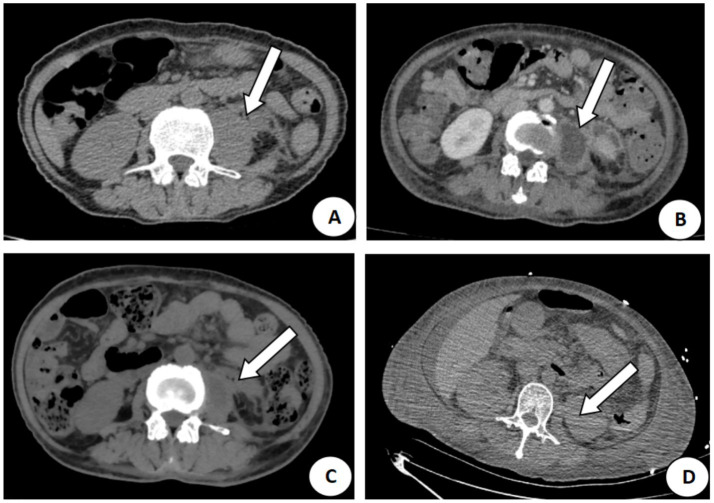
CT images of the lumbar major muscle of the patient in Case 2. (A) Swelling of the left lumbar major muscle, arrows show the extent of inflammation on June 30, 2021; (B) Abscess formation with a diameter of about 2.8 cm on July 9, 2021; (C) Reduction of the abscess to 1.2 cm, arrows show the residual pus cavity on July 23, 2021; (D) Complete resorption of the abscess, and arrows show the normal psoas major muscle structure on January 26, 2024.
